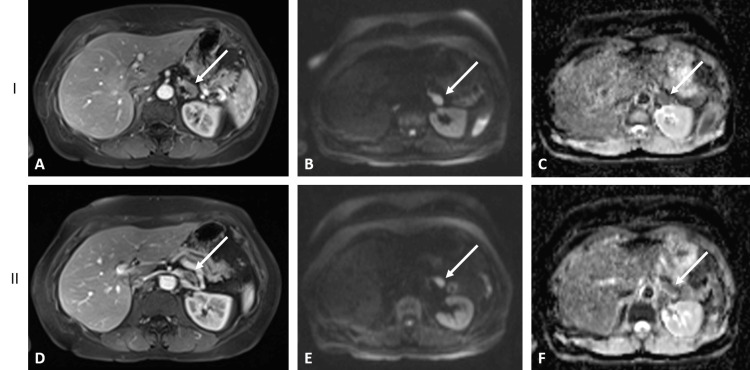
The MRI showing the adrenal metastases (arrows) marked as I and II A and D: Axial T1 fat saturation (FS) after contrast; B and E: Diffusion weighted (B1000); C and F: Apparent diffusion coefficient (ADC)
